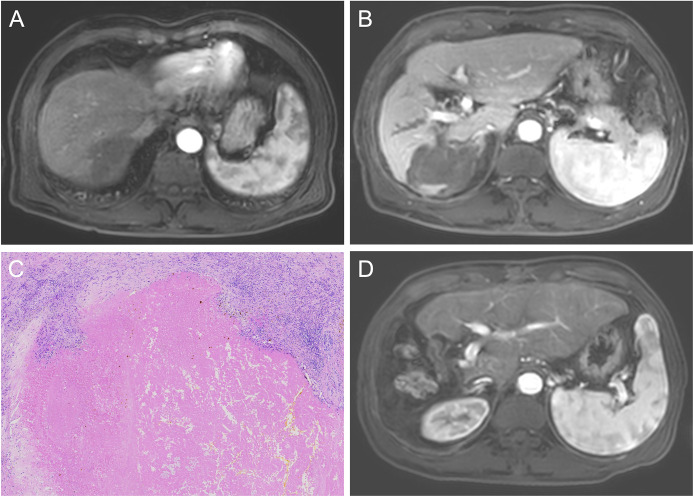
(A, B) Enhanced MR image 26 weeks post-bHAIC shows significant regression of the liver lesion, with complete disappearance of lesion enhancement and resolution of the tumor thrombus in the right portal vein branch. (C) The patient underwent radical resection. Postoperative pathology showed no viable tumor cells, consistent with complete pathological response. (D) Enhanced MR image at 5 weeks post-resection revealed that there was no residual tumor.
